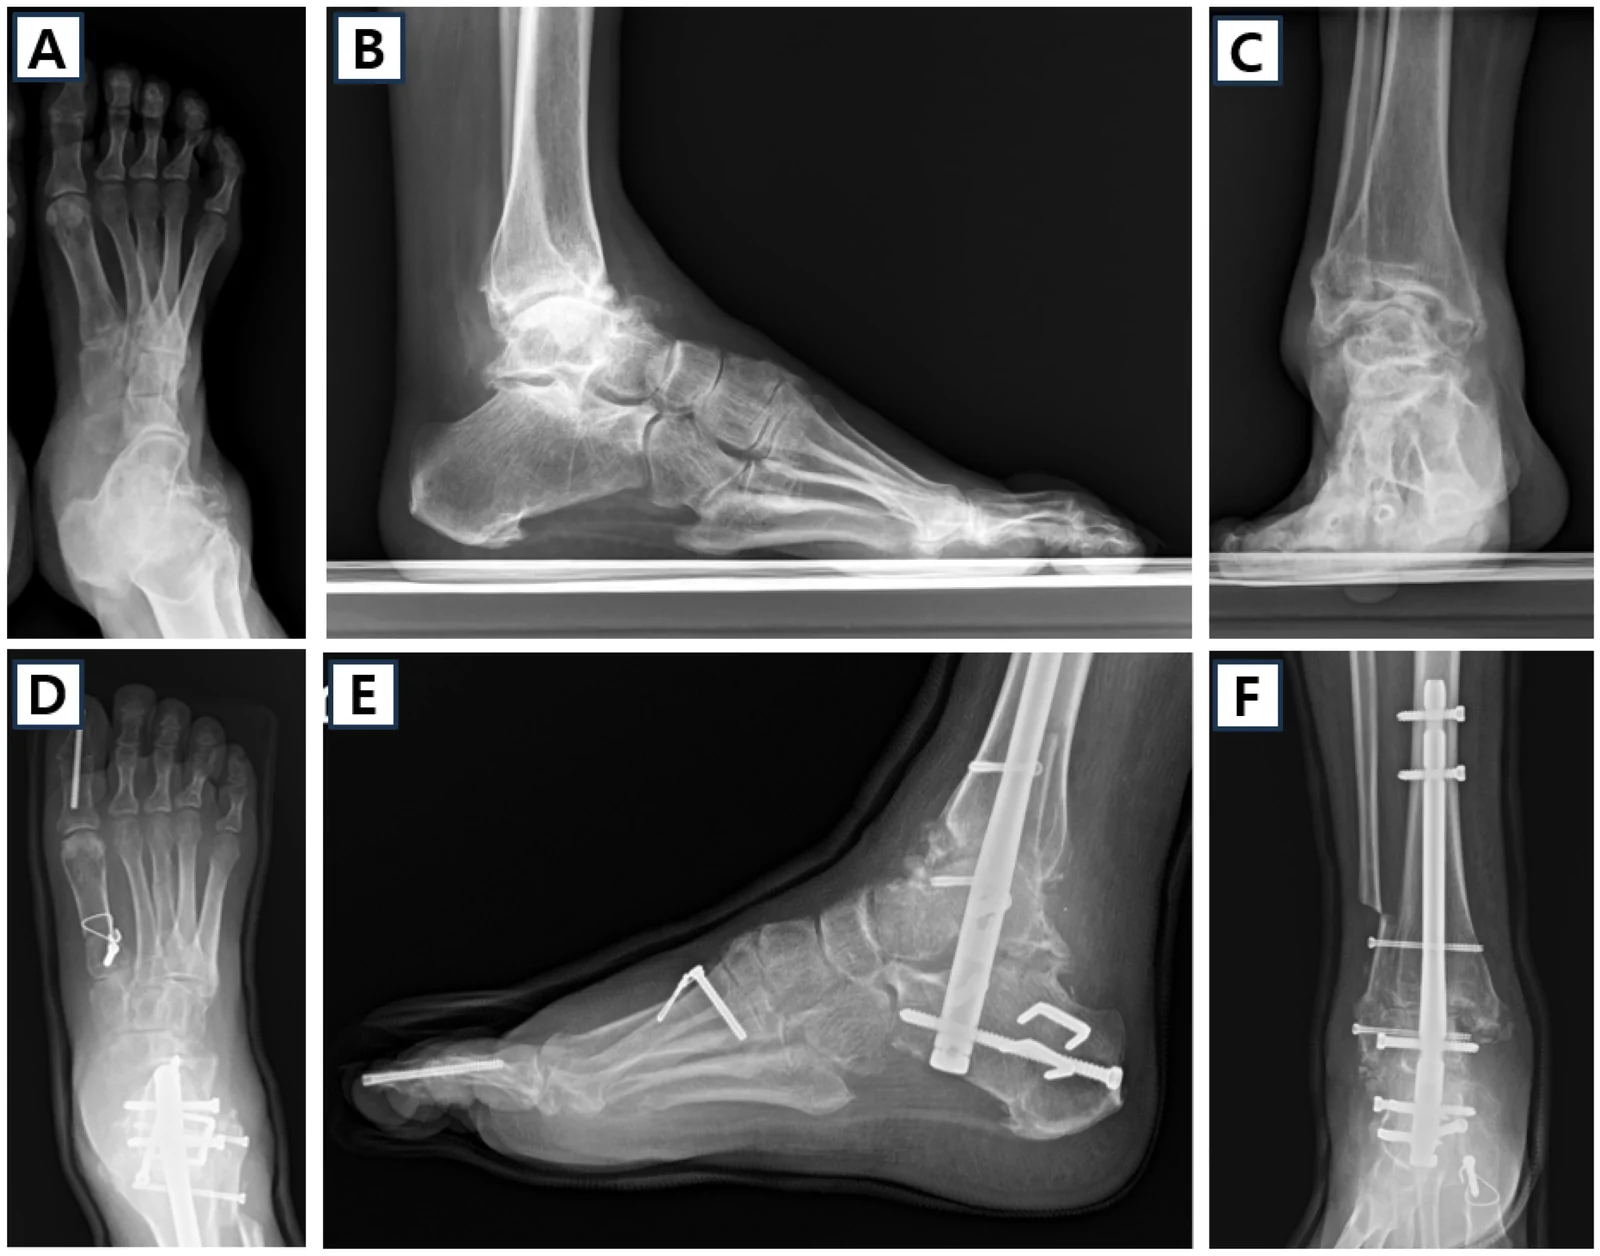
Preoperative and postoperative radiographs of the right foot and ankle in a 40-year-old woman with CMT who underwent joint fusion surgery. Compared with (A–C) the preoperative radiographs, (D–F) the postoperative radiographs demonstrate restoration of neutral foot and ankle alignment following arthrodesis.
